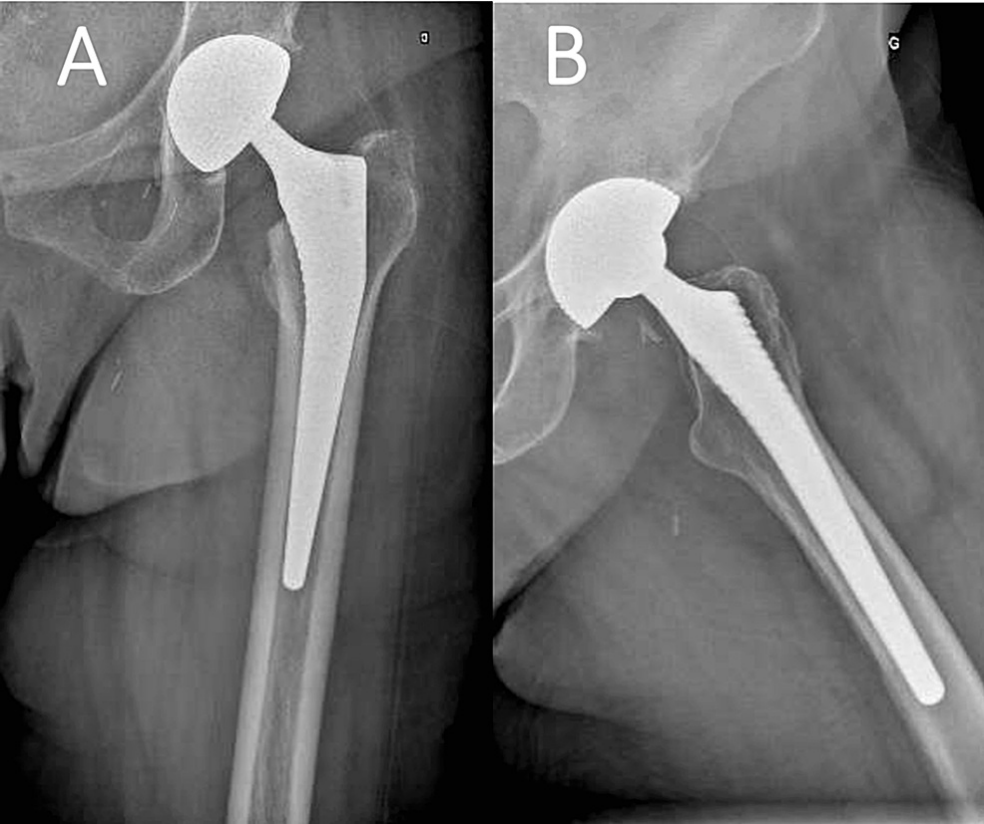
Radiograph obtained six months postoperatively. Standard anteroposterior (A) and lateral (B) radiographs of the operated hip at six months postoperatively.
